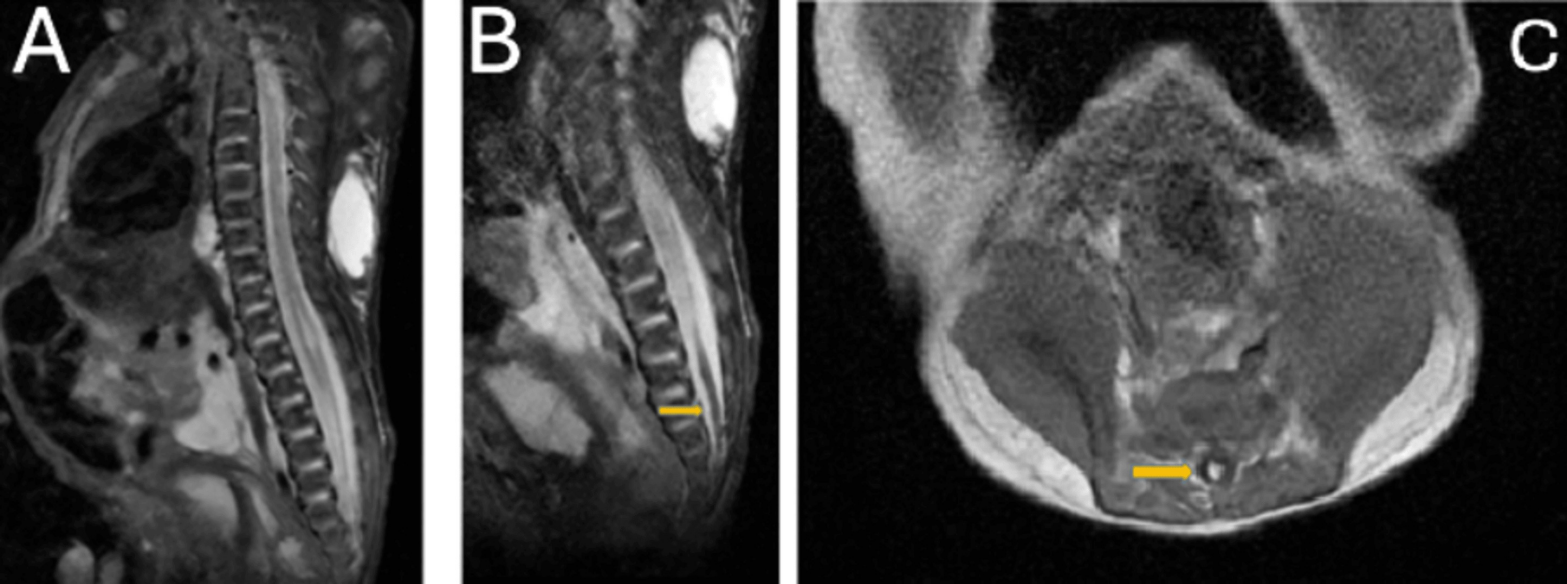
Day 10 post natal MRI centered on the anomalies of the spinal cord A and B: Sagittal T2 fat-saturated views of the syringomyelic cavity of the spinal cord in the lumbar region and an attached cord with lipoma of the filum terminale (arrow). C: Axial T1 view of the filum terminale lipoma (arrow).
